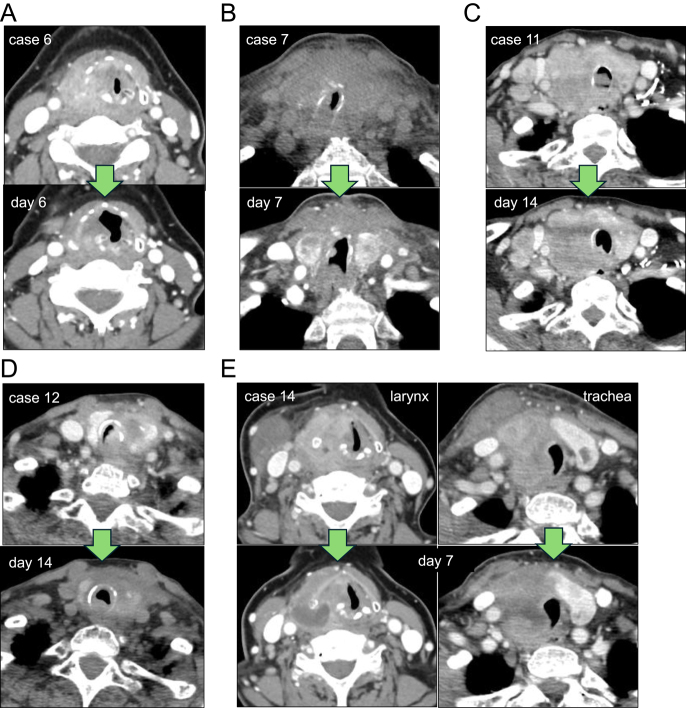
Efficacy of LEN in patients with stridor caused by tumor invasion on the first CT after LEN initiation. Four patients (cases 6, 7, 12, and 14) with ATC and one patient (case 11) with PTC. Airway stenosis was immediately and remarkably improved after LEN initiation in cases 6, 7, 11, and 12 (A, B, C, D), while minor response on airway stenosis was observed in case 14 (E).
